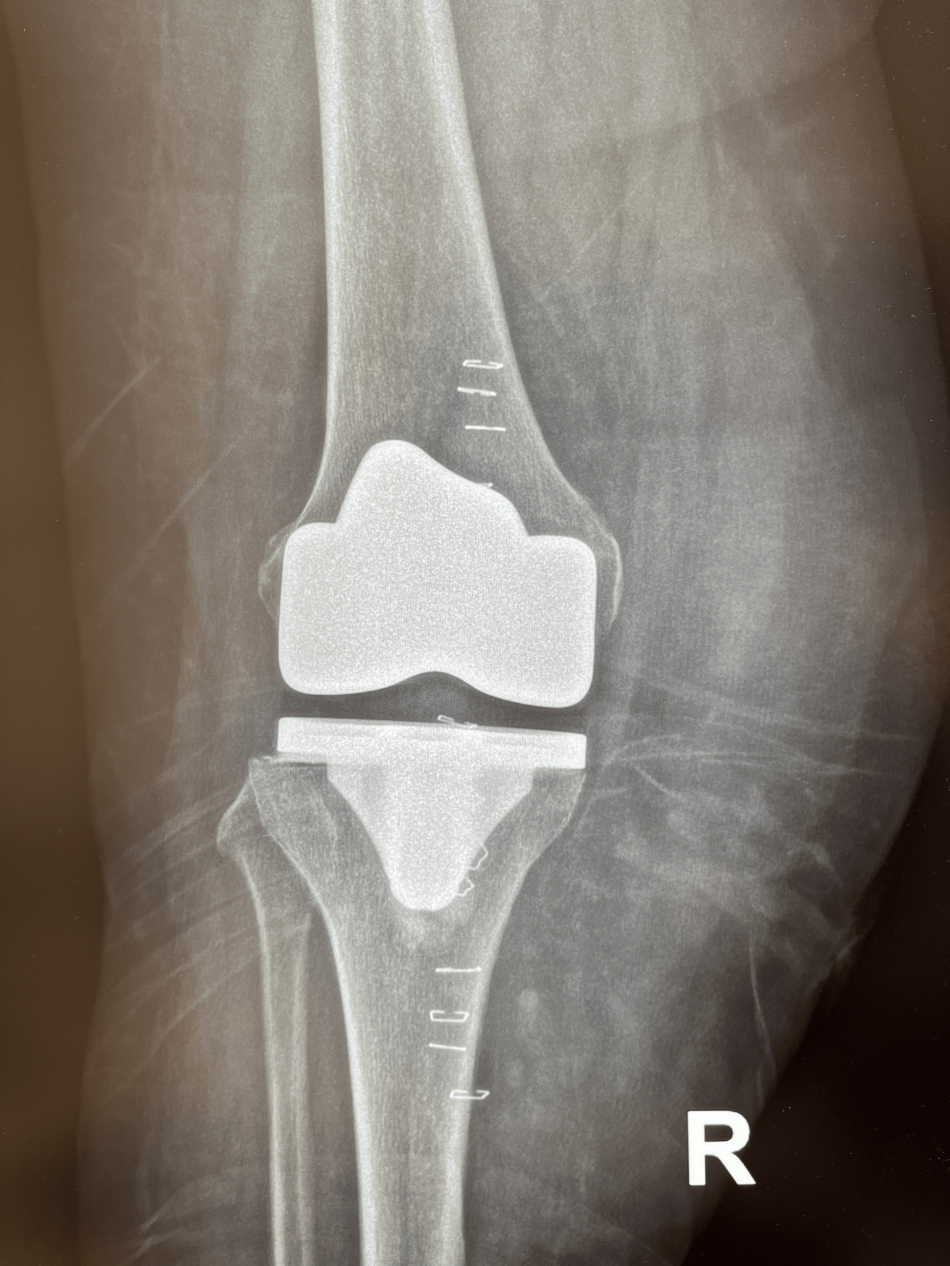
AP (anteroposterior) radiograph of the knee at presentation
